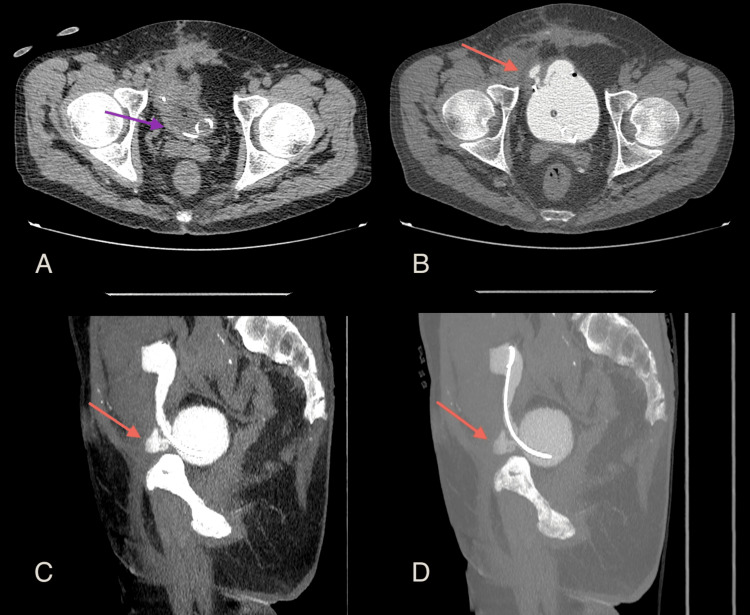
Urine leak of the ureterovesical anastomosis during the second postoperative month of the ureteric reimplantation Multidetector computed tomography (MDCT) urography (axial view) (A) before and (B) after retrograde contrast medium administration; (A) depicts the empty bladder, including the Foley catheter and the distal part of the ureteric stent (purple arrow); (B) indicates a collection of contrast medium outside the bladder at the anastomosis level (red arrow); (C) Sagittal MDCT projection outlines the urine leakage (red arrow); (D) Sagittal MDCT Maximum Intensity Projection (MIP) view depicts the leak (red arrow) and the ureteric stent. Image source: Department of Radiology, Ippokrateio General Hospital of Thessaloniki. Permission obtained for reproduction.
